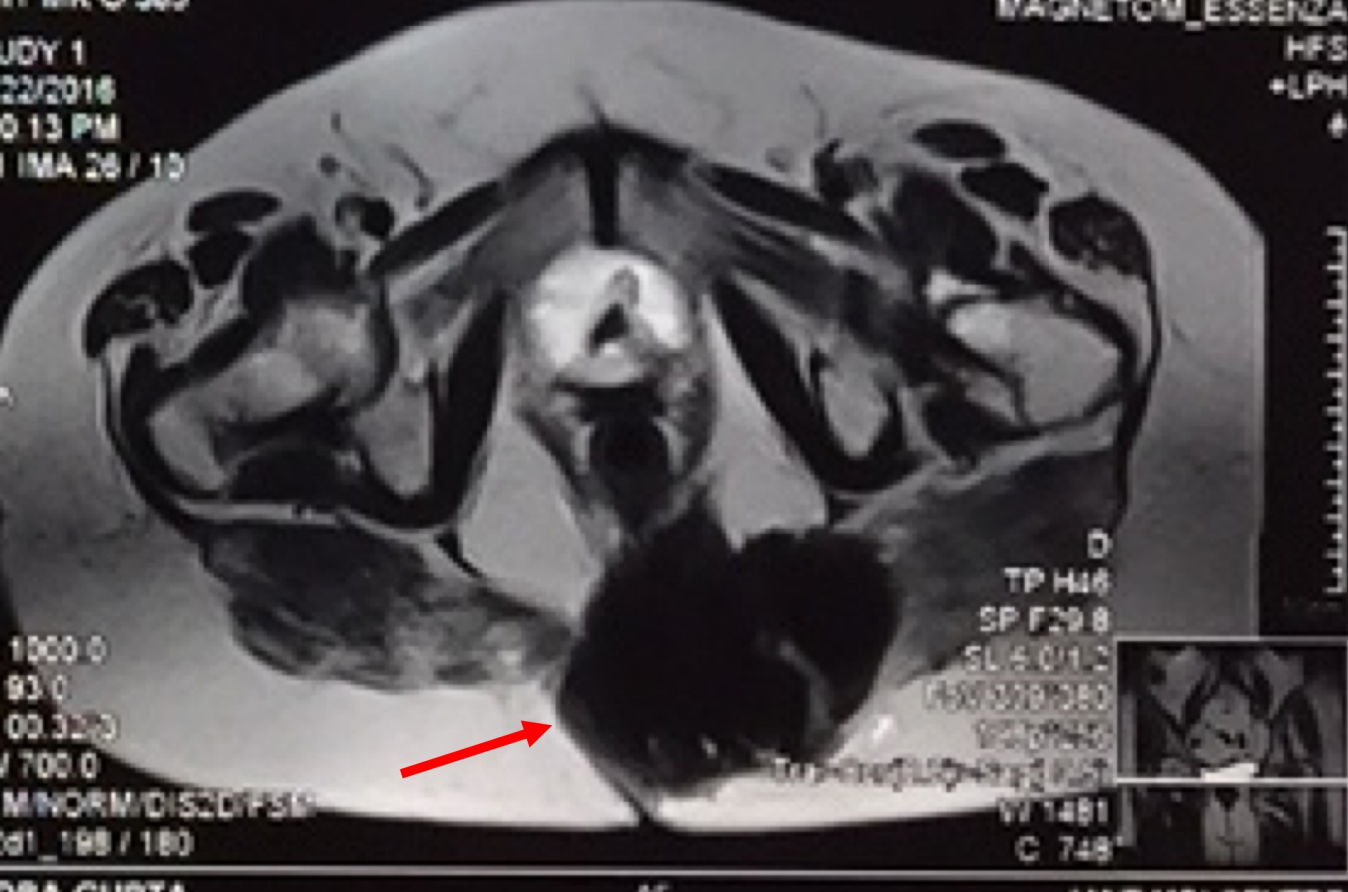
Computed tomography (CT) of the pelvis showing well defined heterogenous lesion in coccygeal region (red arrow) extending into pre- and postcoccygeal compartments in midline and left para midline 84 x 85 x 89 mm in dimension. Few foci of calcifications are noted.
